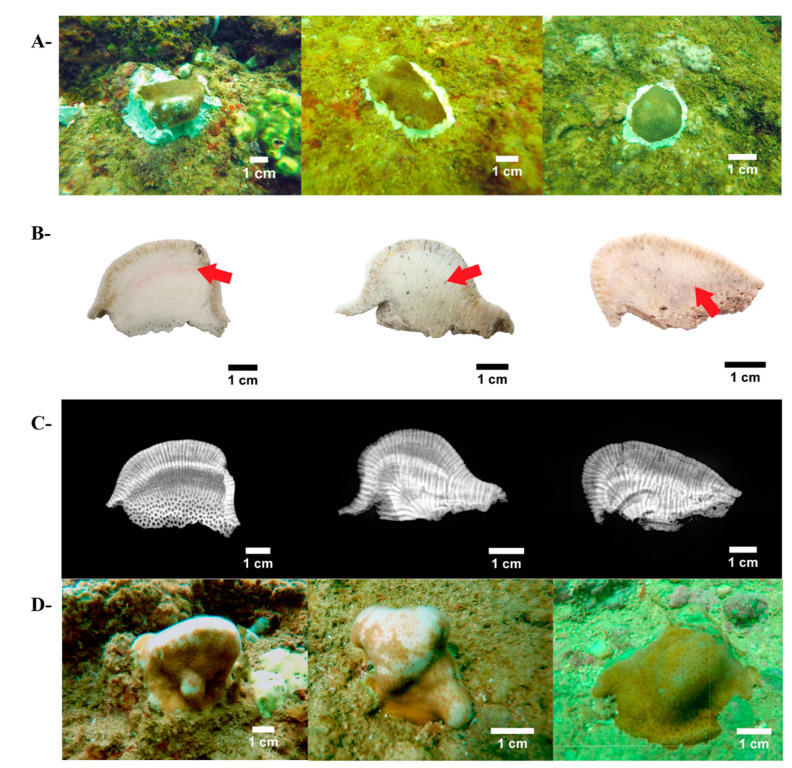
The use of micro-fragments of *Pavona clavus* from Islas Marías Cleofas for coral reef restoration. (A): micro-fragments at onset, just glued to the substrates; (B): coral skeleton slabs exhibiting Alizarin red marks (red arrows); (C): X-ray images of coral skeletons displaying annual bands pattern (light/dark shade = low/high density): (D): final coral size of the same colonies as in part A, 13 months later.
